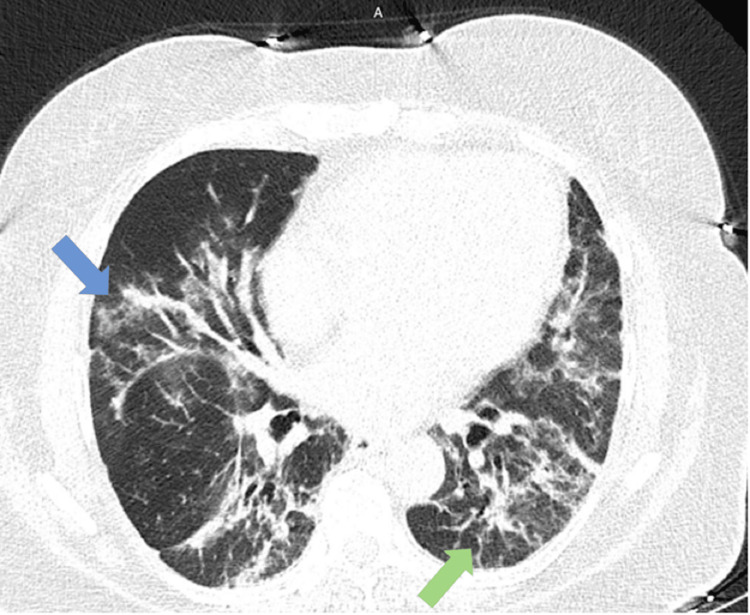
High-resolution CT thorax High-resolution CT (HRCT) after methylprednisolone 125 mg every 6 hours x 7 days and prednisone 60 mg x 4 days. Ground glass opacities (blue arrow) and reticular opacities (green noted) were noted. Features are consistent with cryptogenic organizing pneumonia or nonspecific interstitial pneumonia.
